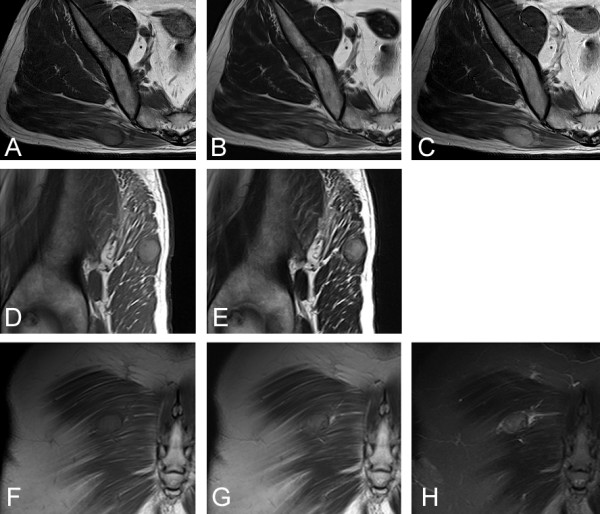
MRI shows diffuse high-signal intensity on T1- (A, D, F; TR/TE = 487/9.5) and T2- (B, E, G; TR/TE = 3500/89) weighted images with capsulation of low-signal intensity on T1- and T2-weighted images (A, B; axial, D, E; sagittal, F, G; coronal). Edema of low-signal intensity on T1-weighted and high-signal intensity on T2-weighted images can be observed (A, B). The tumor has high-signal intensity on T2-STIR (H; coronal). Moderate enhancement by Gadolinium (0.2 ml/kg, Magnebist, Schering, Germany) is seen on T1-weighted image (C; axial). (Siemens Symphony 1.5T, FOV = 200 × 200 mm, matrix = 256 × 320).
